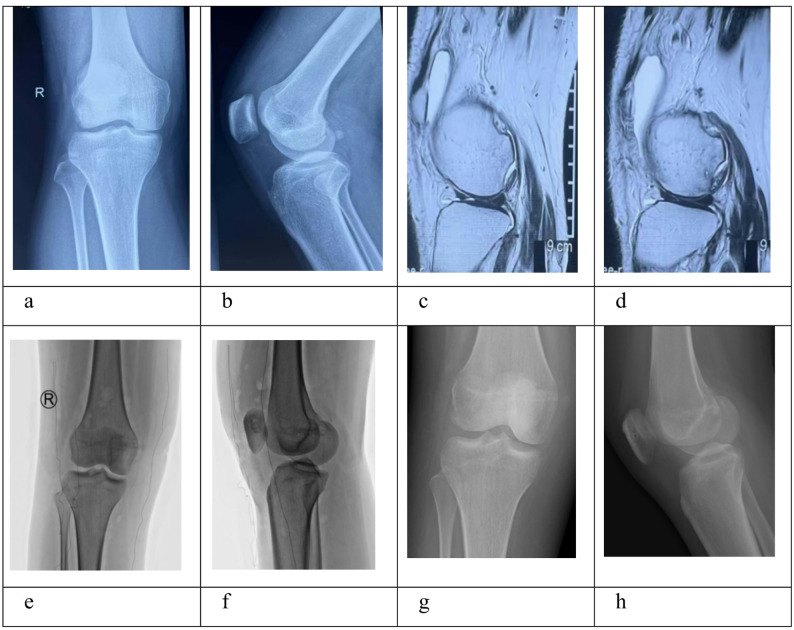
20-year-old female a, b: Preoperative X-ray showing posterior osteochondral fracture of the lateral femoral condyle; c, d: Preoperative MRI showing posterior osteochondral fracture of the lateral femoral condyle; e, f: Postoperative X-ray at 1 day showing OCF fixation achieved; g, h: Anteroposterior radiograph of the right knee at 12 months postoperatively demonstrates good reduction and union of the osteochondral lesion
